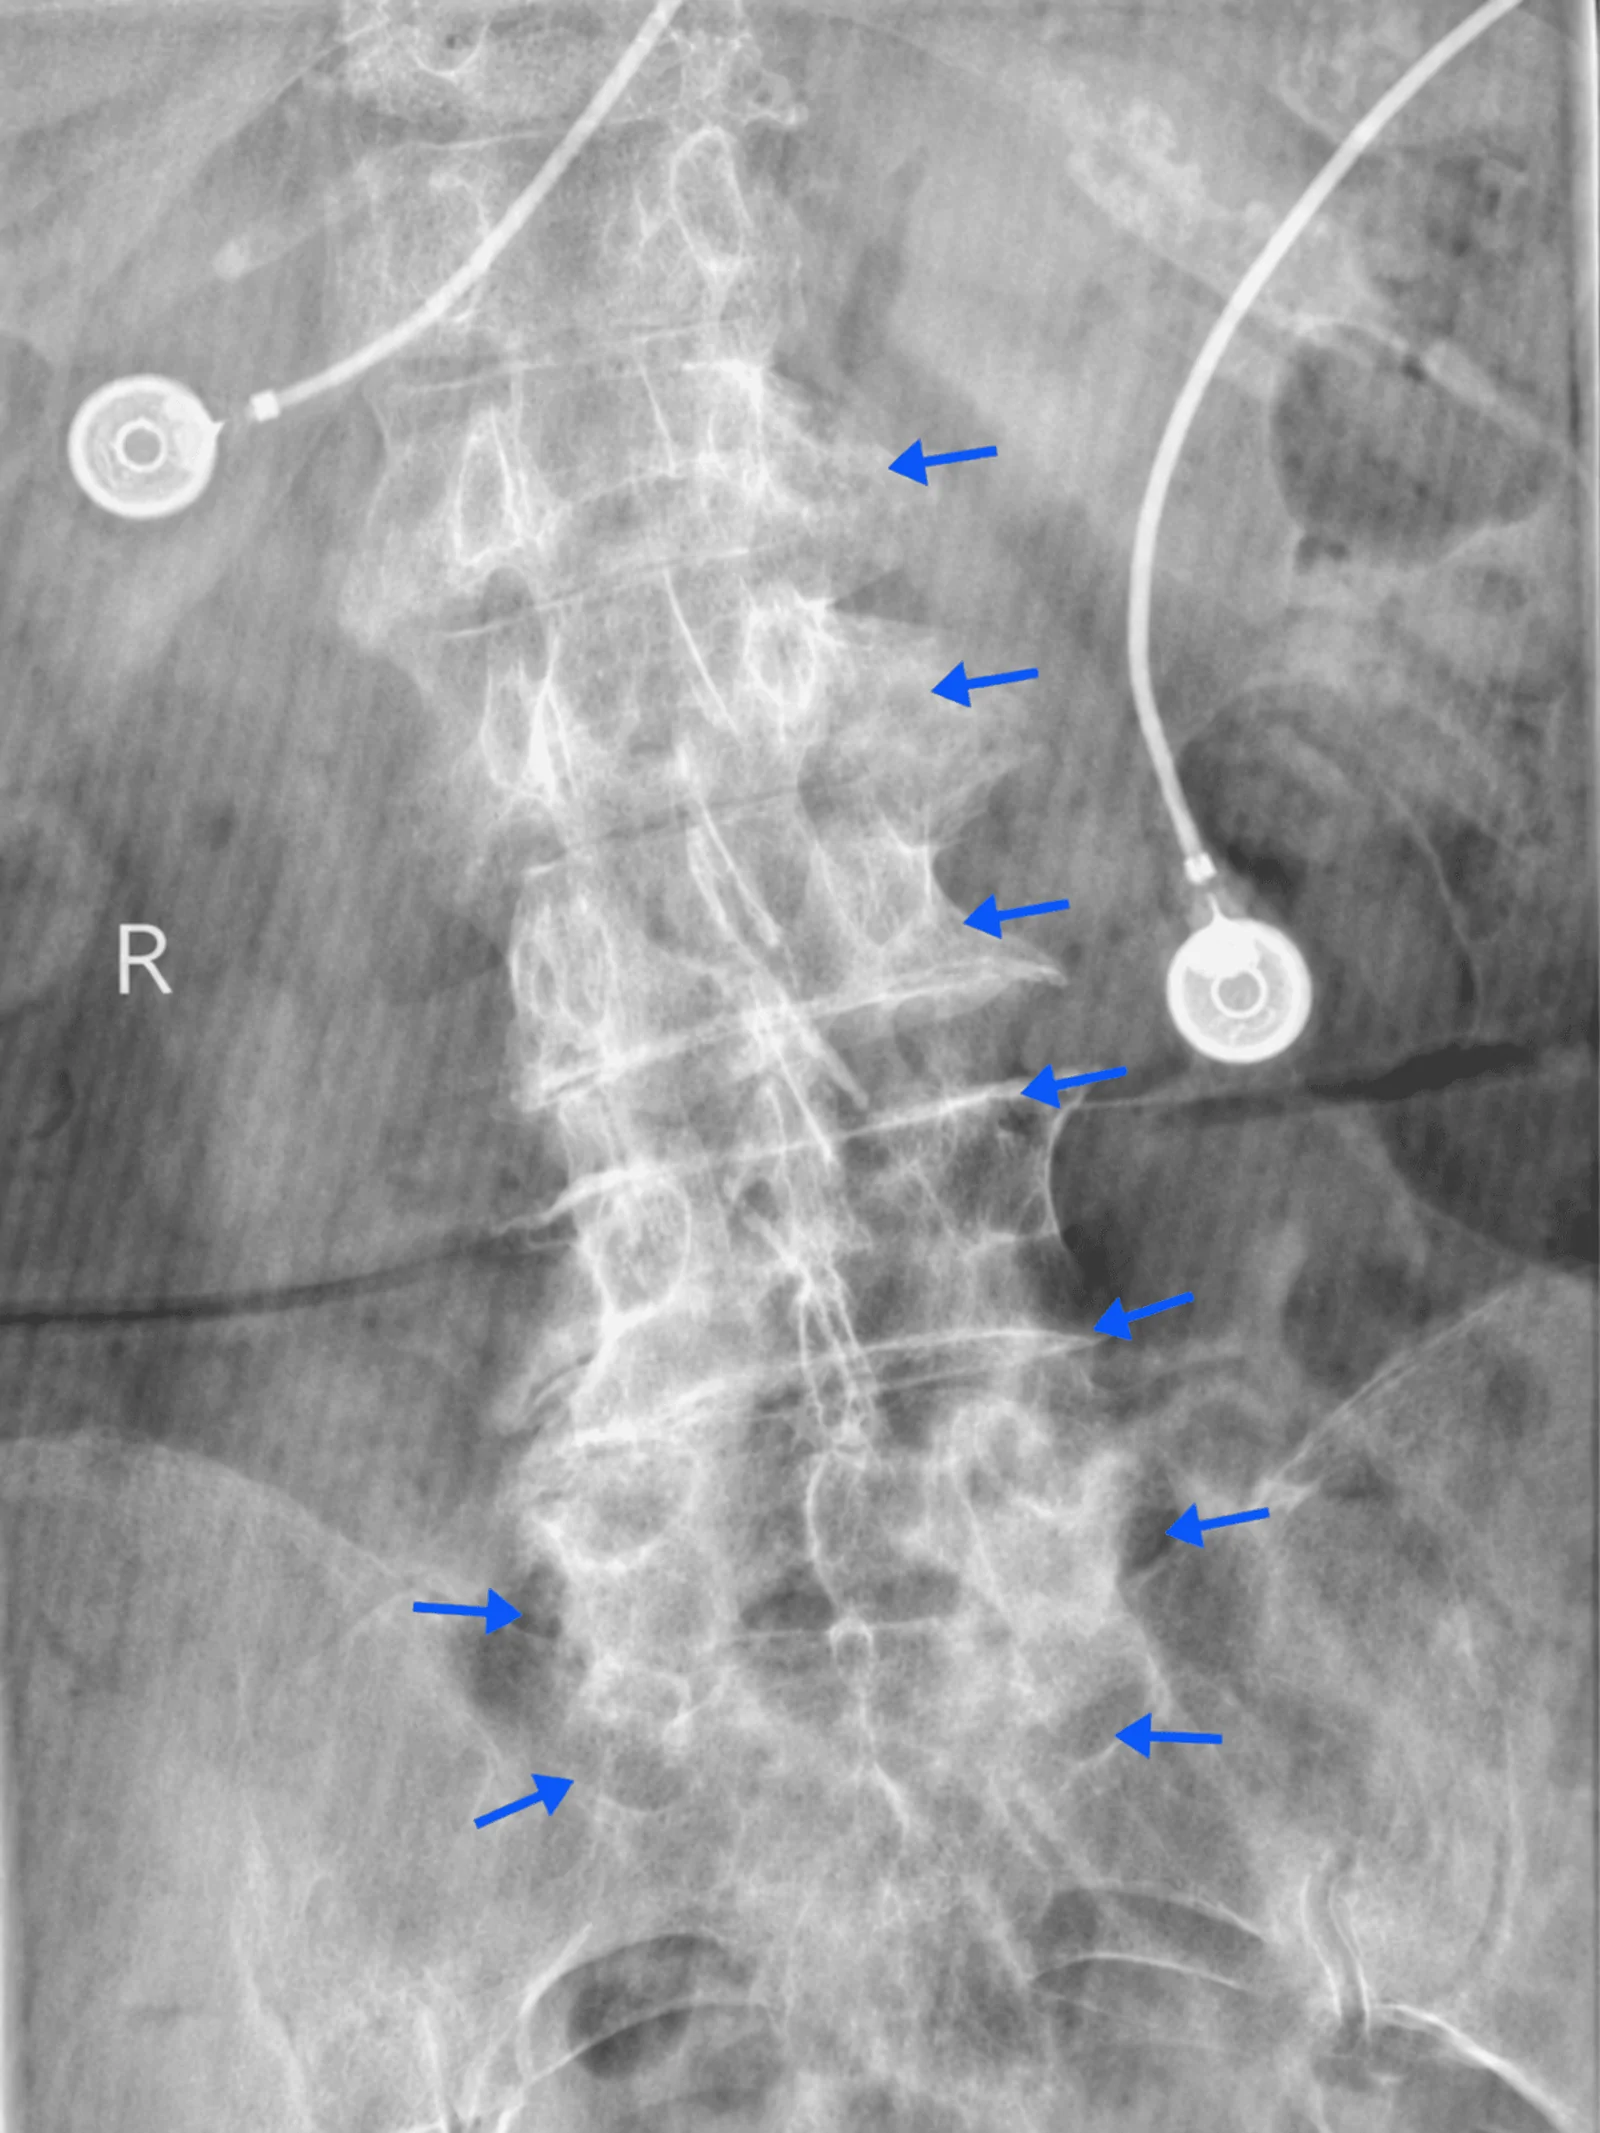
X-ray of the Lumbar Spine X-ray of the lumbar spine showing no acute fracture or subluxation and moderate multilevel degenerative changes of the lumbar spine (blue arrows).
